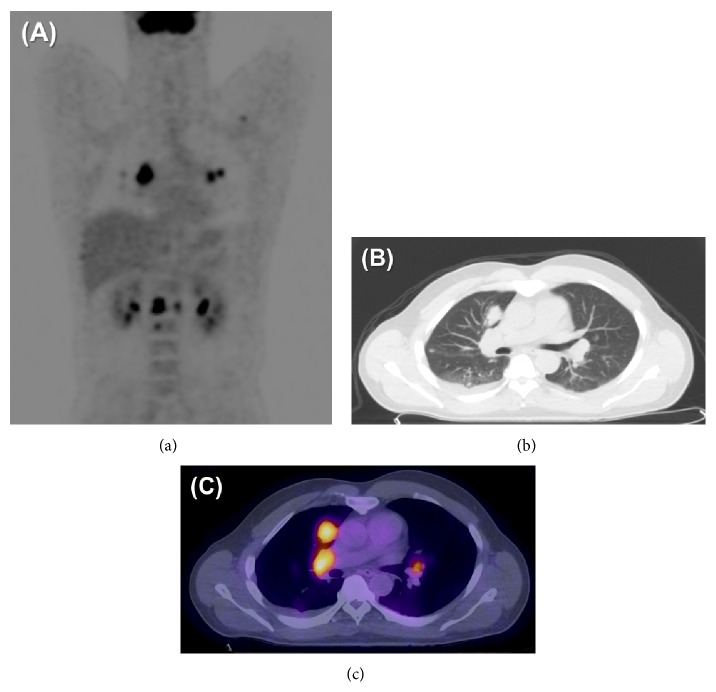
(a) Whole body FDG PET imaging demonstrating hypermetabolic osseous and visceral metastases including bilateral pulmonary nodules and hilar adenopathy. (b) CT imaging demonstrating mediastinal adenopathy, multiple pulmonary nodules, and right pleural effusion. (c) Coregistered PET/CT imaging demonstrating intense FDG uptake within GBM lung metastases.
